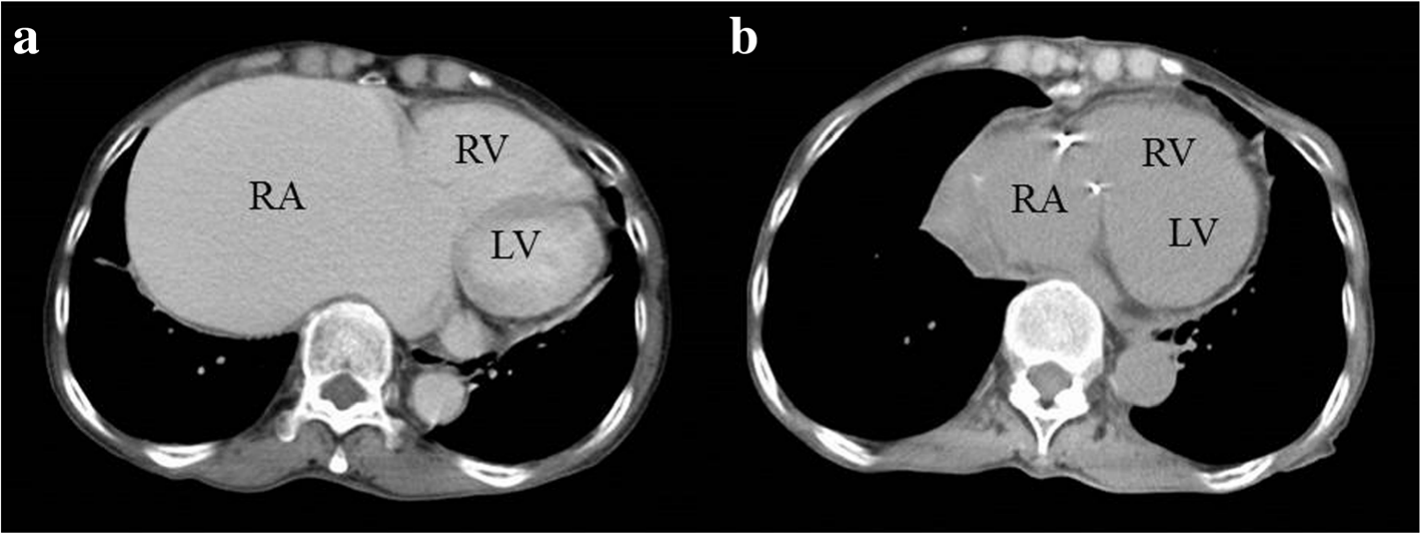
a Computed tomography (CT) findings showed that the size of the RA increased gradually up to 121 mm 1 year previously. b Postoperative CT scan showed reduction of the RA. LV, left ventricle; RA, right atrium; RV, right ventricle
